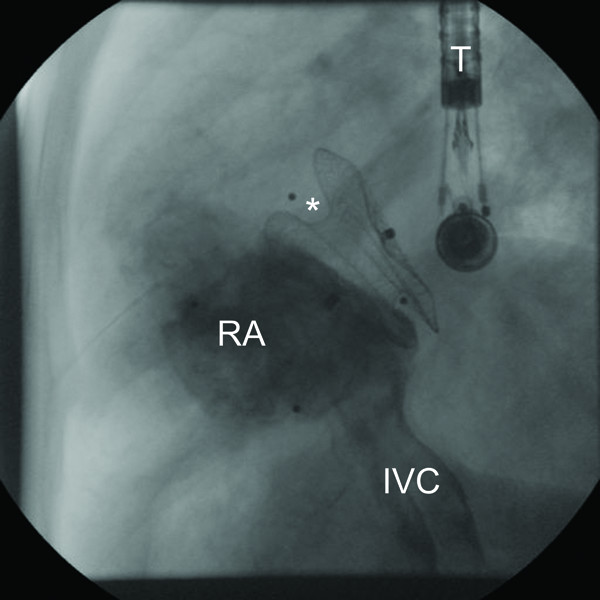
Lateral fluoroscopy after interventional closure of ASD: Contrast media is injected in the IVC showing the right atrium. Amplatzer Occluder in position of former ASD bracing from aortic root (asterisk). T: transesophageal probe; IVC: inferior vena cava; RA: right atrium.
